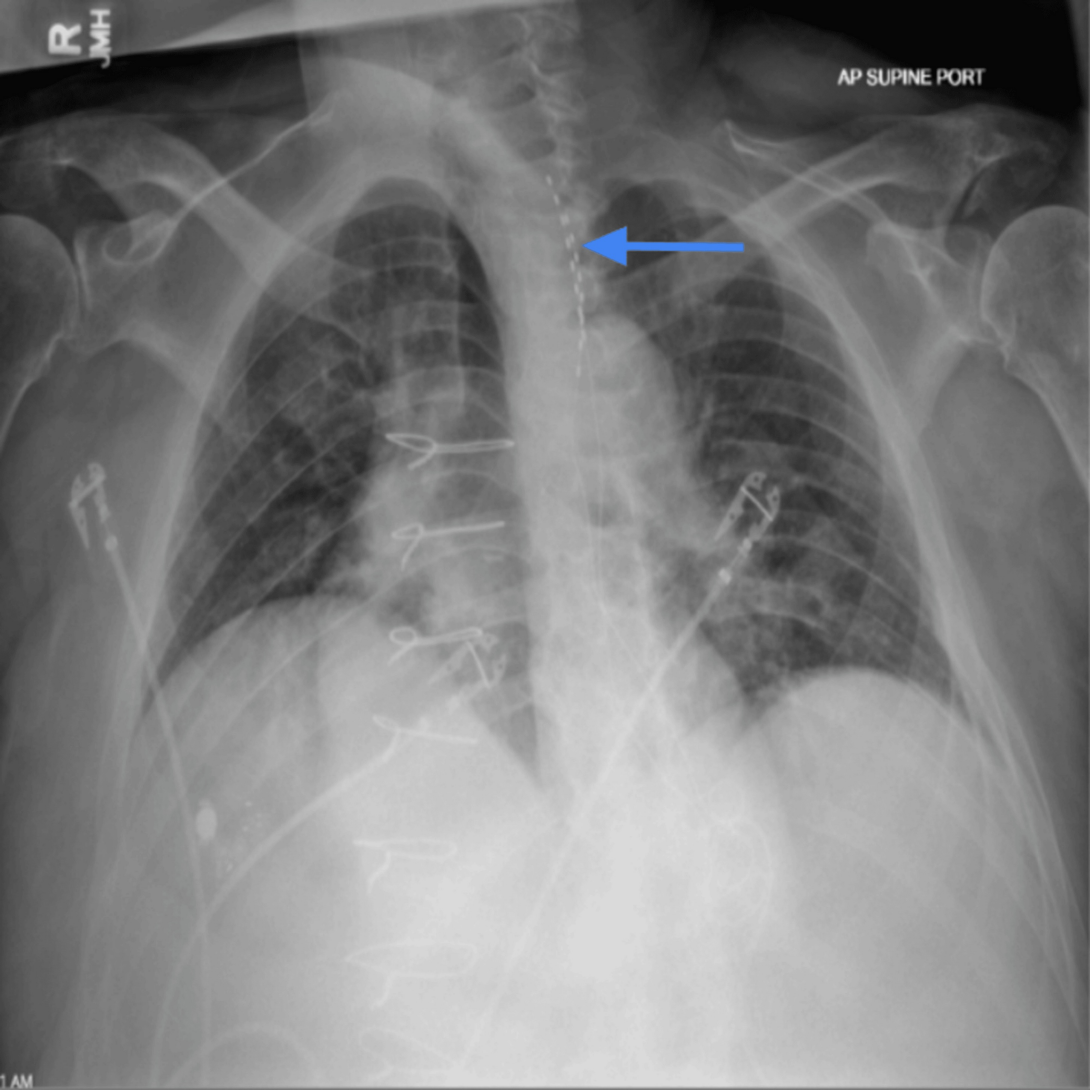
Anteroposterior chest radiograph obtained during the spinal cord stimulation trial demonstrating the projected location of the percutaneous leads (arrow) over the upper thoracic spine. Mild rotational artifact may have limited precise assessment of strict midline orientation on this view alone. Posterior epidural location and final lead positioning were confirmed intraprocedurally using orthogonal fluoroscopic imaging.
